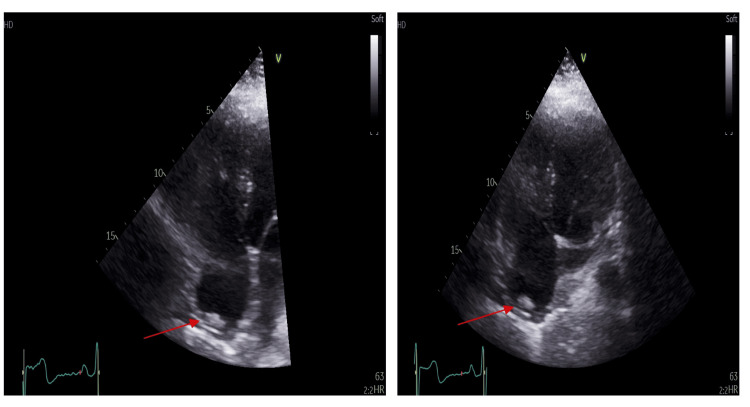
Transthoracic echocardiography demonstrating a right atrial mass. Apical four-chamber view showing a well-defined echogenic mass within the right atrium (red arrow).
